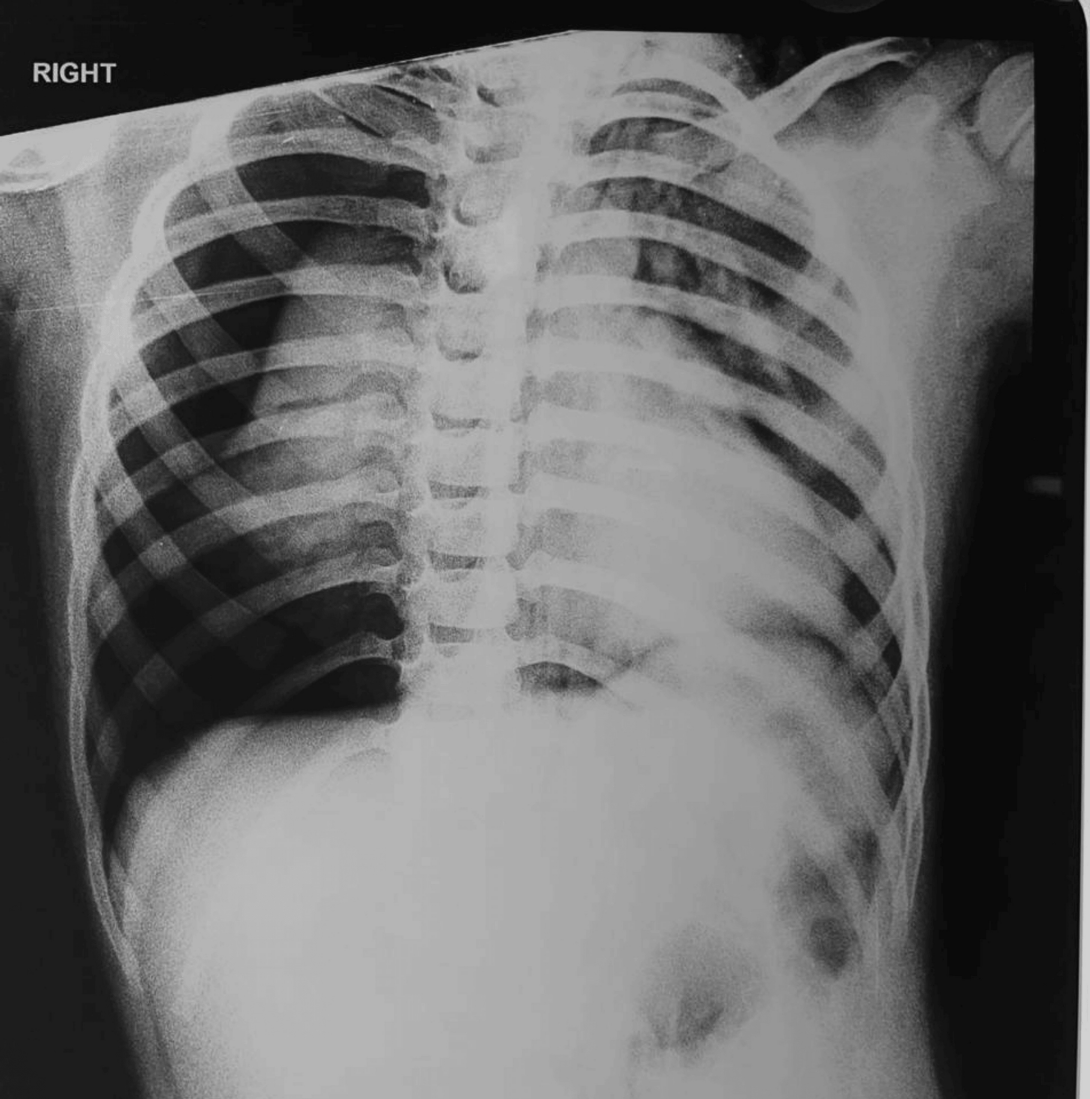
Intraoperative chest X-ray showing a large right-sided pneumothorax and a small left-sided pneumothorax.
